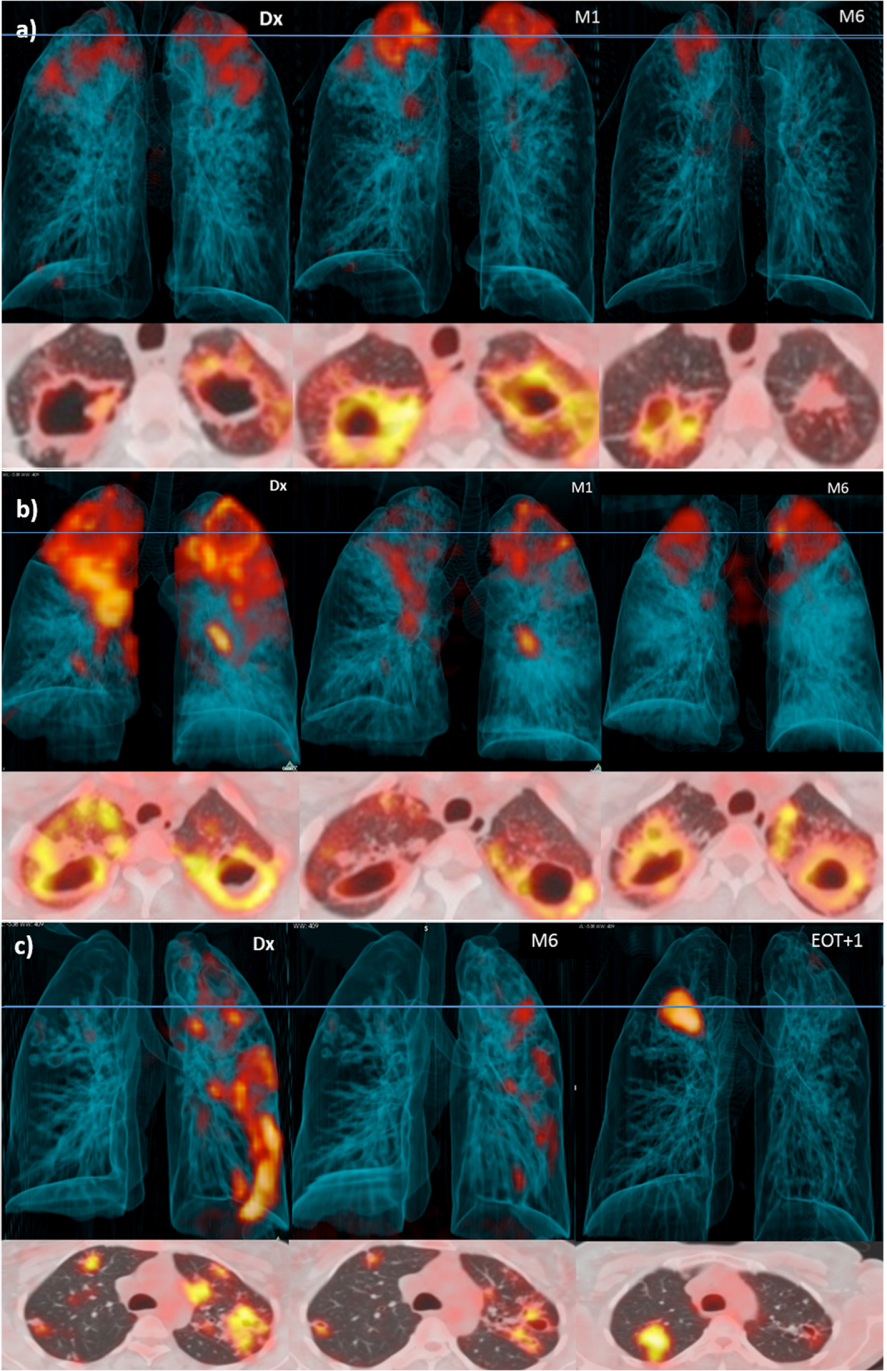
FDG PET-CTs for three representative cases that received 6 months of standard treatment. Three-dimensional anterior and transverse slices at the level of horizontal blue line. a Bilateral upper lobe cavitation at Dx, which demonstrate increased intensity at M1. At M6, the left cavity has changed to fibrotic tissue with mild uptake, but the right cavity still has a thick wall and high uptake. b Failed treatment case with bilateral upper lobe cavities that retain very high intensity at M6. c Case diagnosed with recurrent disease subsequent to EOT + 1y scan. All lesions improved to moderate intensity uptake at M6, but a large new area of patchy consolidation is seen at EOT + 1y
